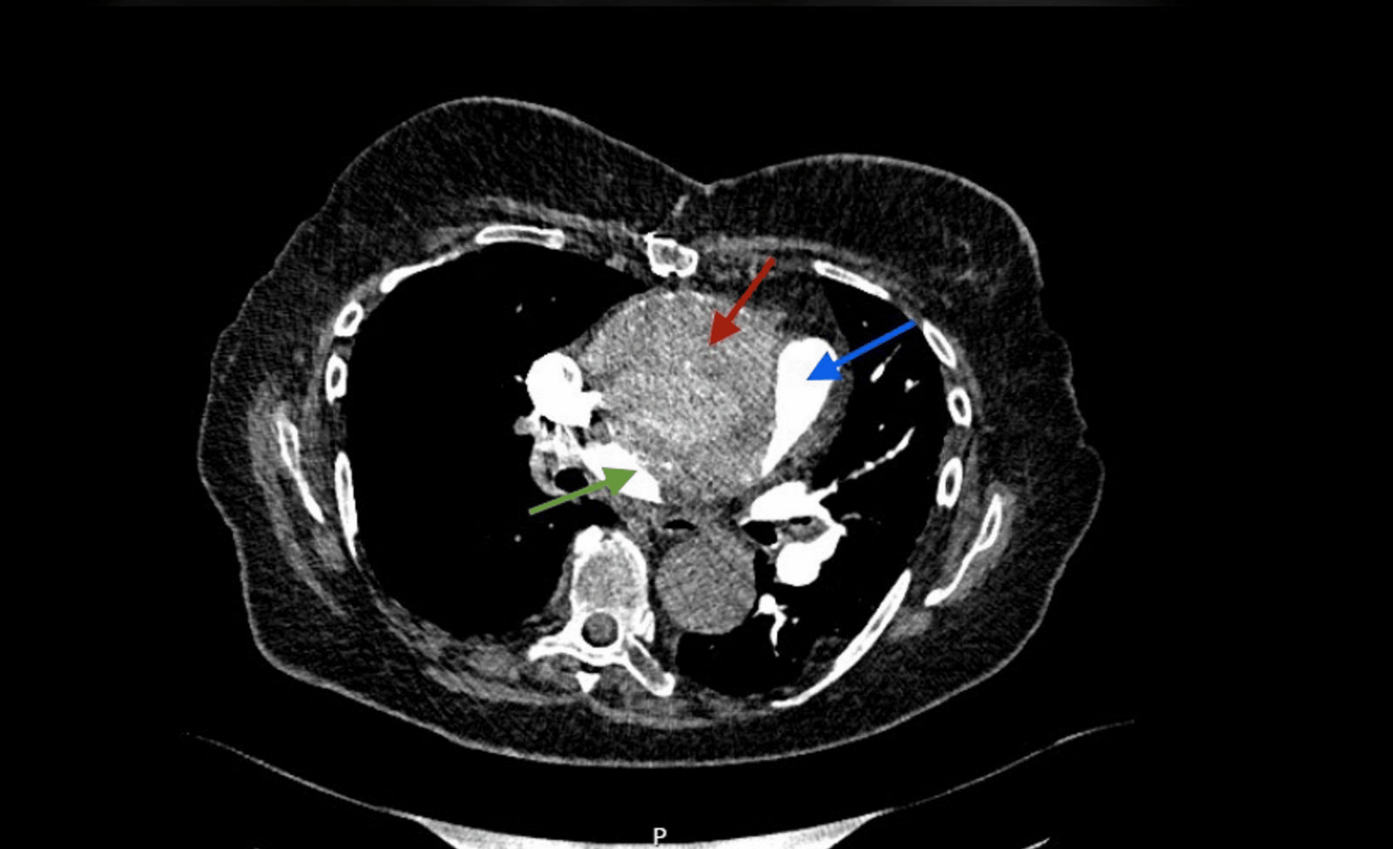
Computed tomography pulmonary angiogram (CTPA) CTPA showing an increase in size of the pseudo-aneurysm ventrally. The diameter of the ascending aorta has increased to 67 mm (was 58 mm). There is a thrombus in the pulmonary trunk and the right main pulmonary artery, resulting in marked narrowing of the residual lumen. The red arrow shows the aneurismatic ascending aorta. The blue arrow shows the pulmonary trunk. The green arrow shows the right main pulmonary artery with thrombus.
